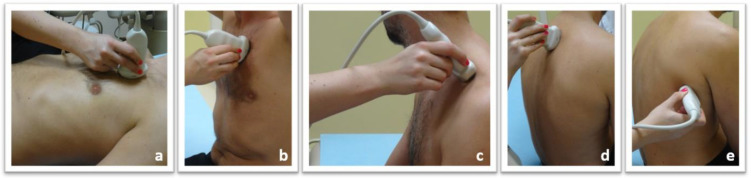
Lung ultrasound technique for internal medicine patients: Lung ultrasound examination in patients with internal diseases is performed with the convex and linear probe, in the supine and/or sitting position: (a) Scanning the anterior chest wall in the supine position; (b) Scanning the anterior chest wall while seated; (c) Assessment of the peaks through the supraclavicular fossa; (d) Assessment of the posterior chest wall in a sitting position; (e) Assessment of the posterolateral thoracic wall in a sitting position.
